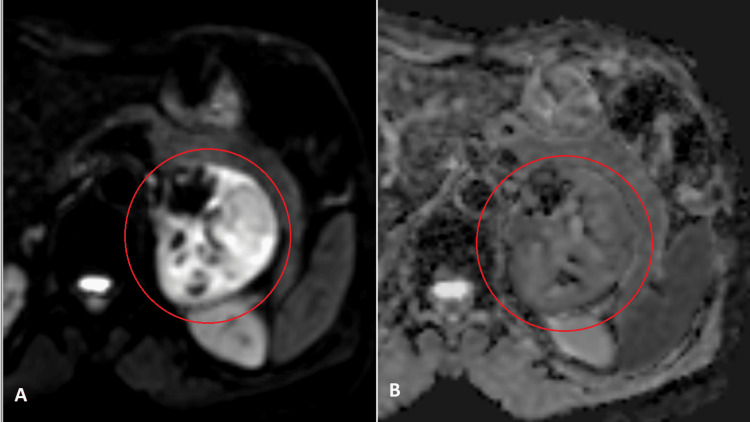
Axial diffusion-weighted imaging study, abdominal MRI. Left adrenal solid mass (circles) with diffuse and intense hypersignal on diffusion-weighted imaging for b values of 1000 (A) and corresponding diffuse moderate hyposignal on ADC map, showing restricted diffusion.
